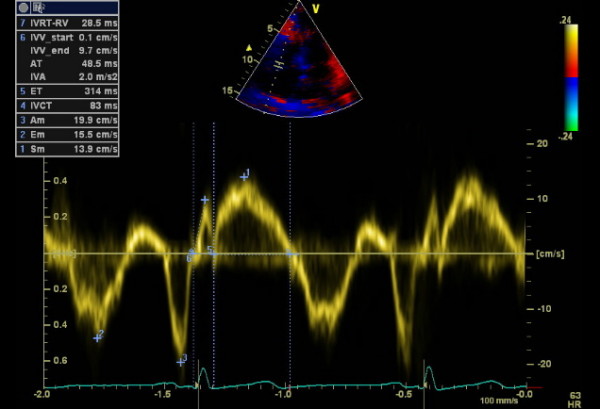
Pulsed wave tissue Doppler imaging of the RV free wall of an SSc patient. 1: peak myocardial systolic velocity (Sm), 2: peak early diastolic velocity (Em), 3: peak late diastolic velocity (Am) 4: isovolumetric contraction time (IVCT), 5: ejection time (ET), 6: peak myocardial isovolumetric contraction velocity (IVV), acceleration time (AT), isovolumetric acceleration (IVA), 7: isovolumetric relaxation time (IVRT-RV).
